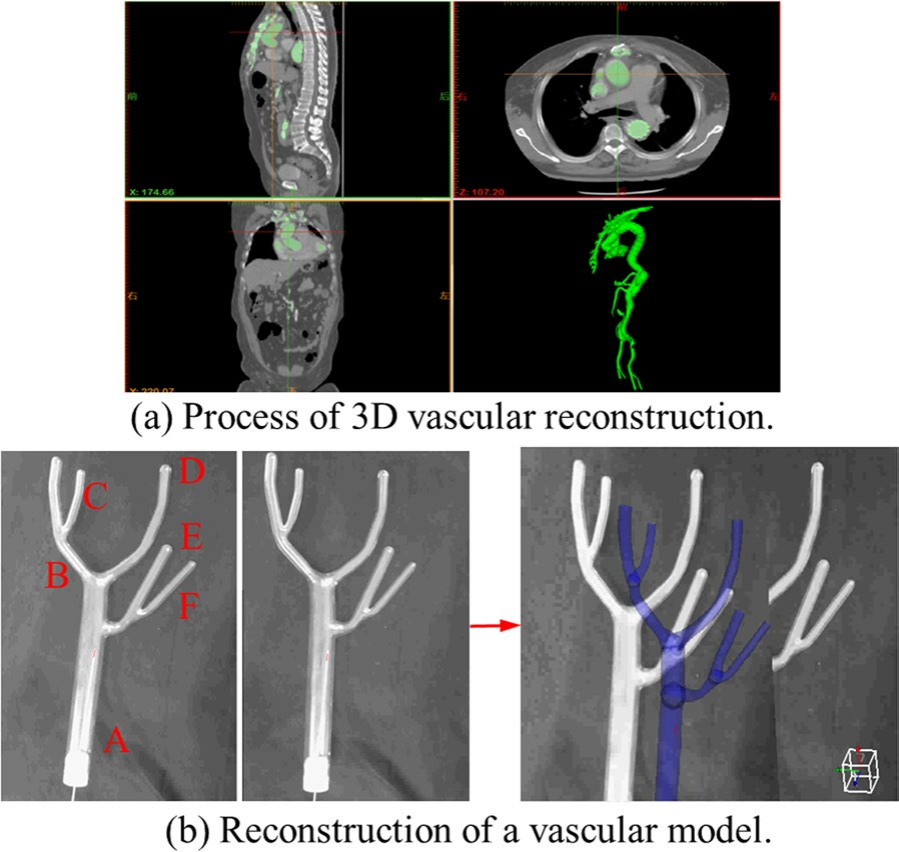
3D reconstruction of CTA slices and the vascular model
